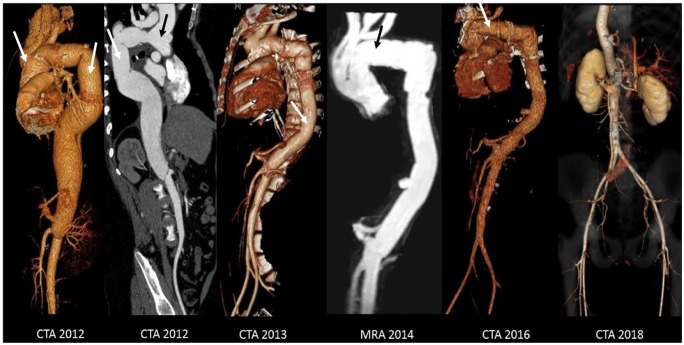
Computed tomography angiography and magnetic resonance angiography serial follow-ups. Various procedures were performed before or after shown scans: Bentall procedure (2005) and descending thoracic replacement (2011); thoracoabdominal replacement (2013); and aortic arch replacement (2014). New surgical repairs are denoted by arrows.
